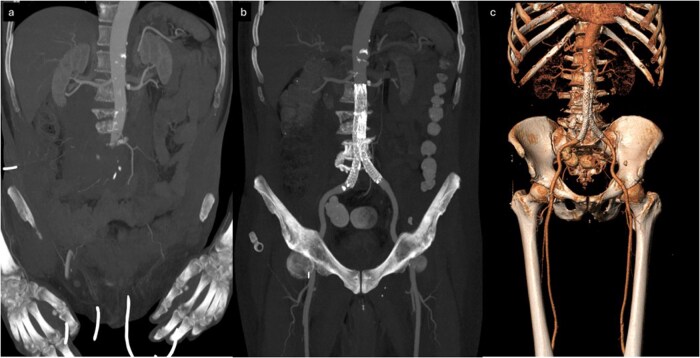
Coronal MIP reconstructions. (A) Initial CT at admission shows absence of opacification of the abdominal aorta distal to the level of L3, with poor visualization of the iliac or femoral arteries. (B) Post-operative CT following endovascular repair with aortic endograft demonstrates restored opacification of the abdominal aorta and excellent visualization of the iliac arteries, including both internal and external branches, as well as the common femoral arteries and their distal runoff. Image in c is a VRT of a follow-up CT at 10 days, demonstrating correct positioning of an aorto-bi-iliac endograft with complete restoration of vascular opacification of the abdominal aorta, its major branches, and the arterial circulation of both lower limbs.
